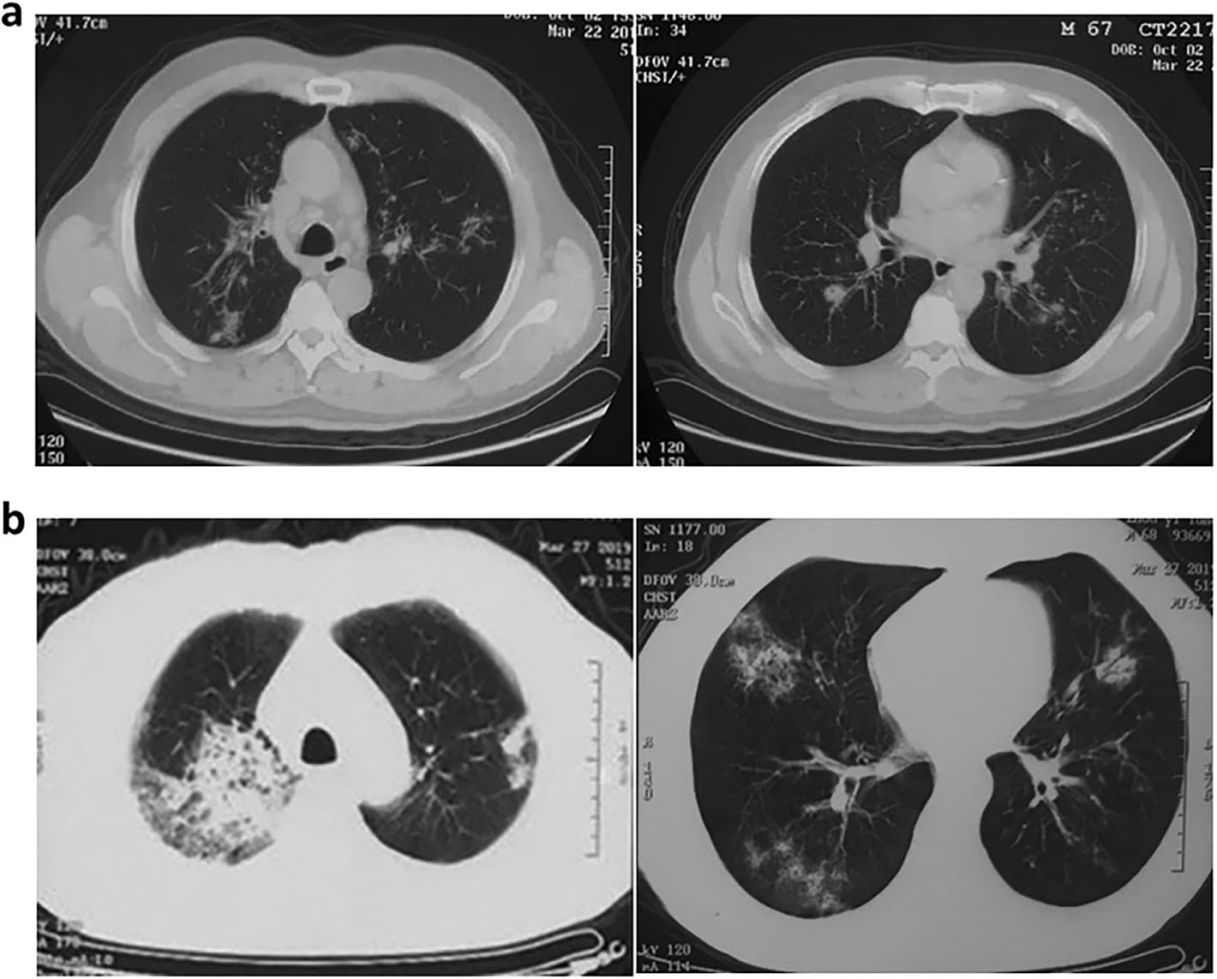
a CT on March 22 showing bilateral diffuse ground-glass opacities and multi-focal, patchy, ill-defined nodular opacities in the lungs. b Newly developed multi-focal dense consolidations are observed
